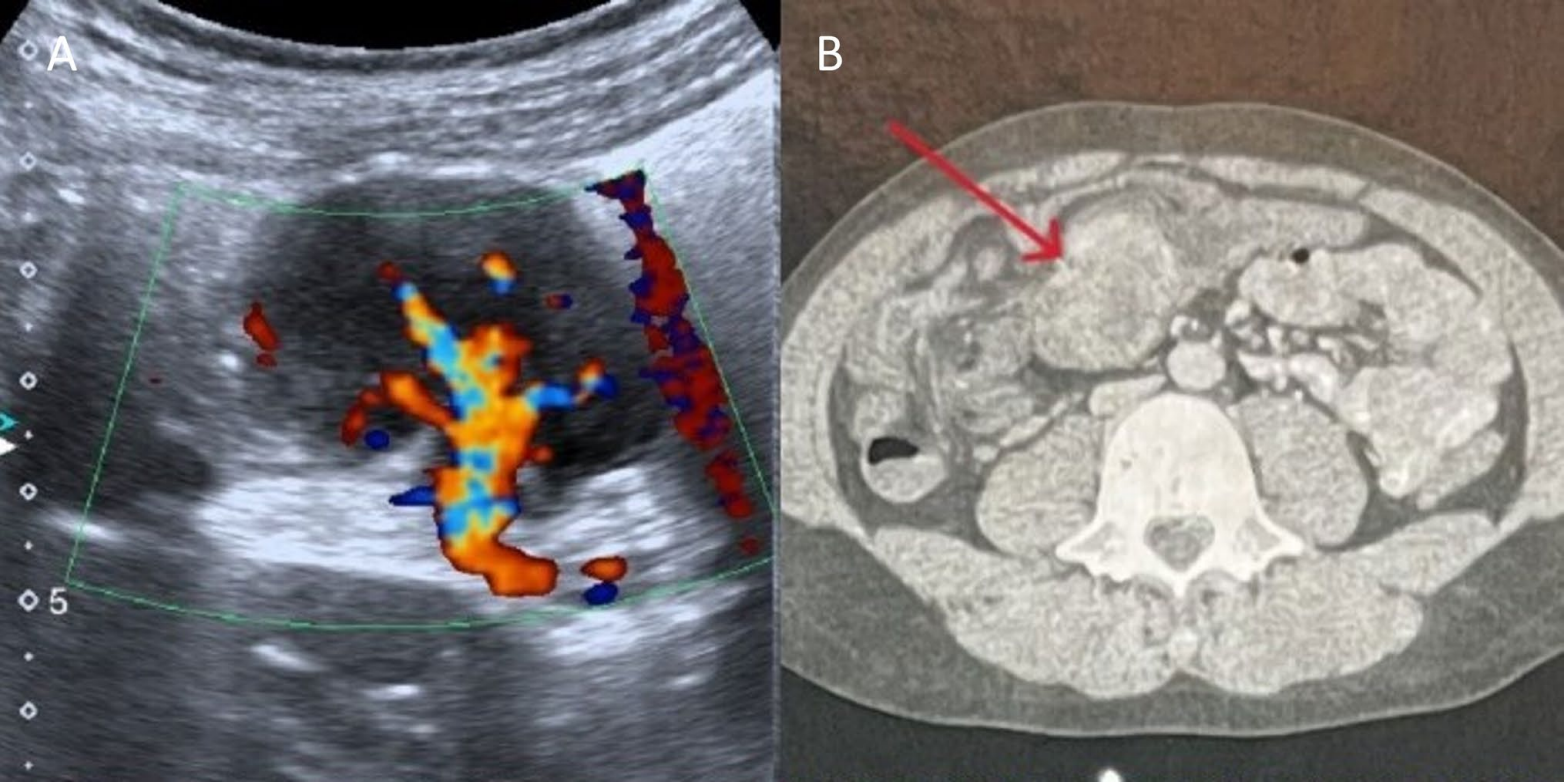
A Abdominal ultrasound view of the tumor showing a highly vascular central pedicle. B Tumor localized at the ascending colon (arrow)
